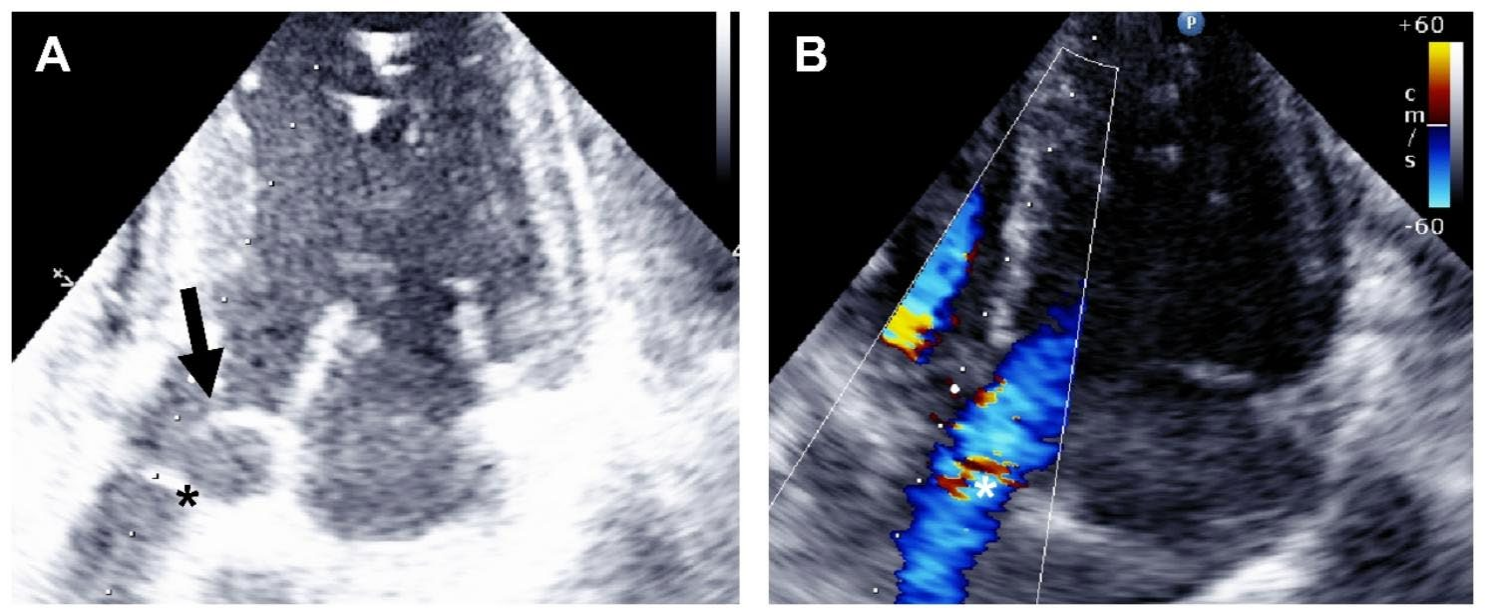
Intra-aortic band in (A) 2D echocardiography and (B) with color flow Doppler. (A) In 2D echocardiography, an intra-aortic hyperechoic structure (*) distal to the aortic valve (arrow) was found at the level of the sinotubular junction. (B) Color flow Doppler displayed unspecific turbulences around this structure
